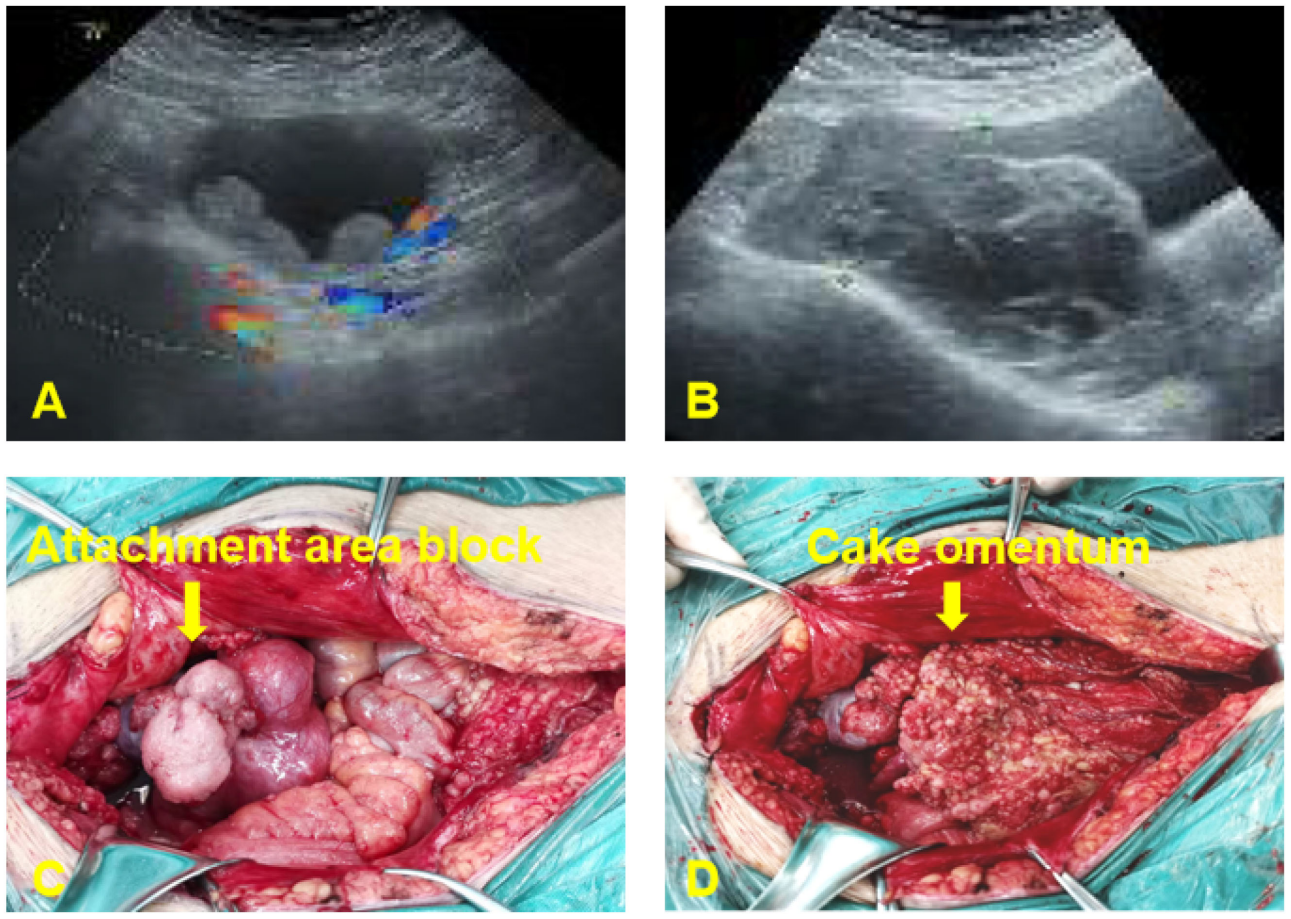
Preoperative ultrasound examination and intraoperative investigation of the patient. (A) Lunar 4, 2021: A 7.0*7.6 cm cystic mass was found in the upper left part of the uterus, with a thick cyst wall and multiple slightly hyperechoic protrusions. (B) May 10, 2022: A 12.8x10.1x5.8 cm solid cystic mass with a detached and multilocular cystic portion was present in the right adnexal region. The mass crosses the anterior aspect of the uterus and involves the left ovary. (C) Exophytic granular masses in the adnexal region bilaterally. (D) Granular metastatic nodules in the greater omentum.
